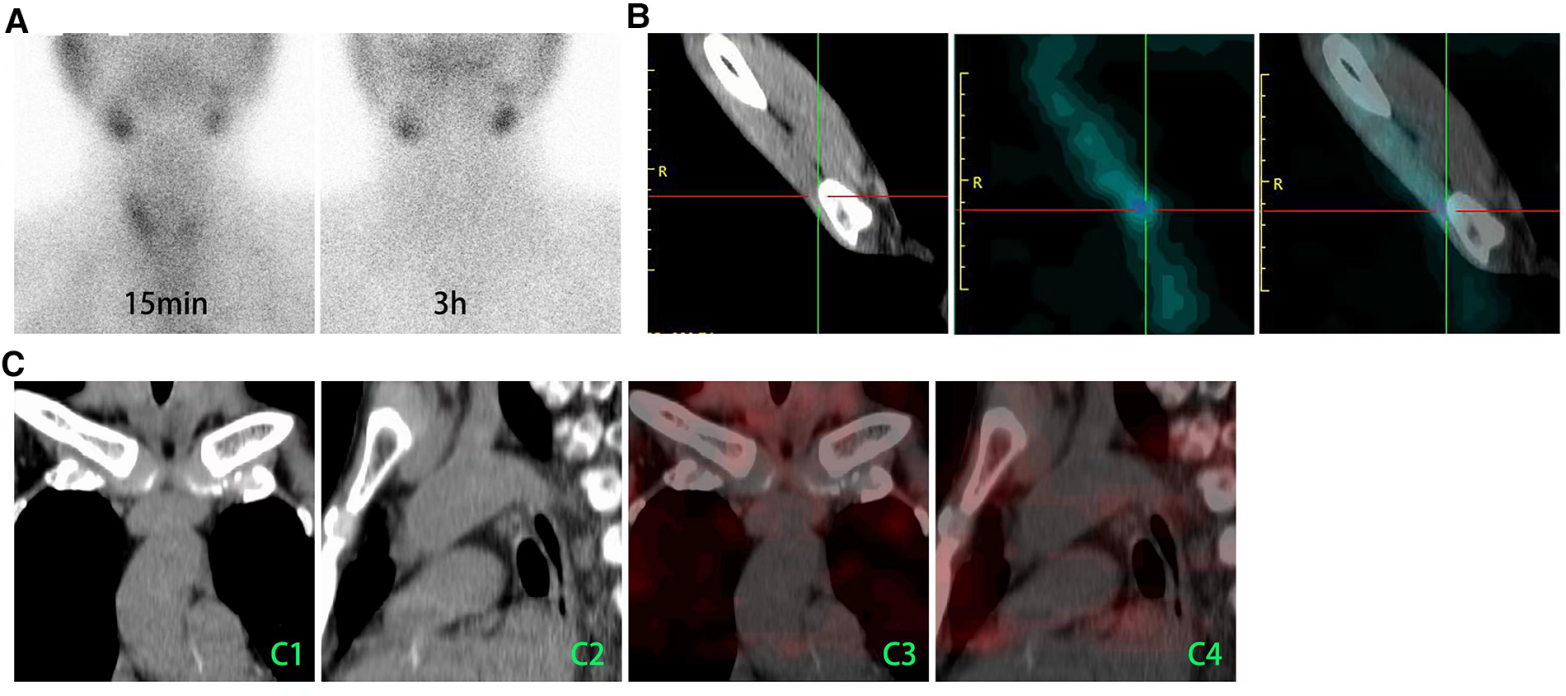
^99m^Tc-MIBI/SPECT was used to demonstrate whether the suspected parathyroid tissues had been missed. (A) ^99m^Tc-MIBI planar early (15 min) and delayed (3 h) static images showed that there is no tissue with focal tracer accumulation that presented in the primary surgery site of neck. (B) A weak focal tracer accumulation had presented in the autograft site. (C) The axial CT (C1 and C22) and SPECT/CT (C3 and C4) images showed no tissue with MIBI uptake in the mediastinum. ^99m^Tc-MIBI, ^99m^Tc-sestamibi; CT, computed tomography.
